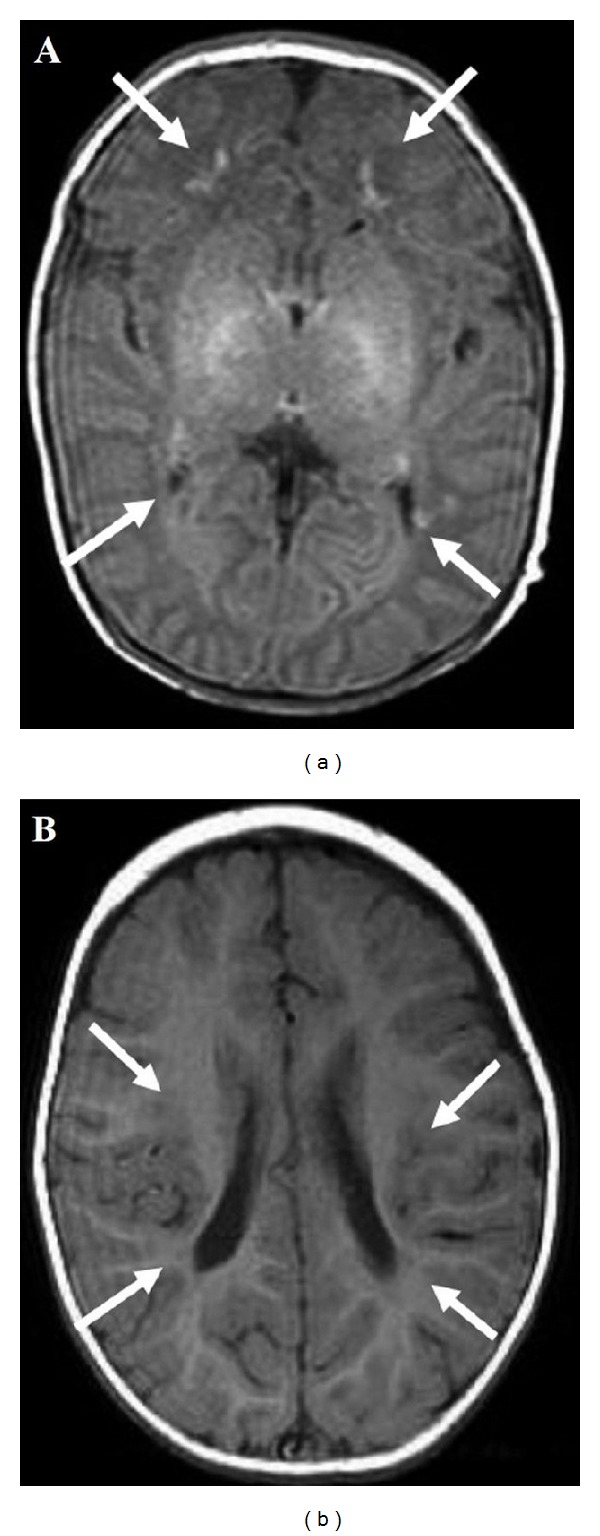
Brain MRI. Newborn with classic adrenal congenital hyperplasia, on day 12 after birth, white matter abnormalities that consisted of bilateral small diffuse hyperintensities (white arrow) are depicted on T1-weighted images (a). At 1 yr. of age, bilateral small diffuse hyperintensities are documented (white arrow) on T1-weighted images (b). (Reprinted with permission from [49], Copyright Japanese Society for Pediatric Endocrinology).
